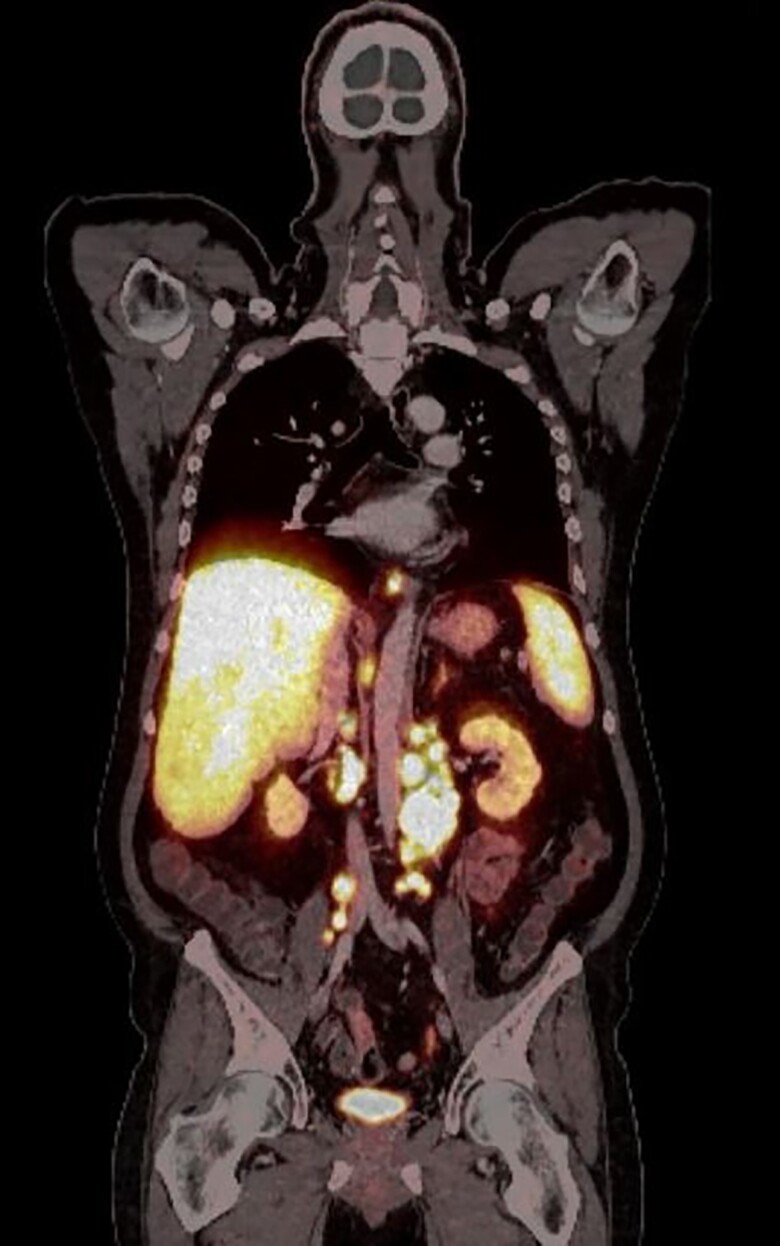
Positron emission tomography–computed tomography revealing lymphatic para-aortic and hepatic metastasis.
